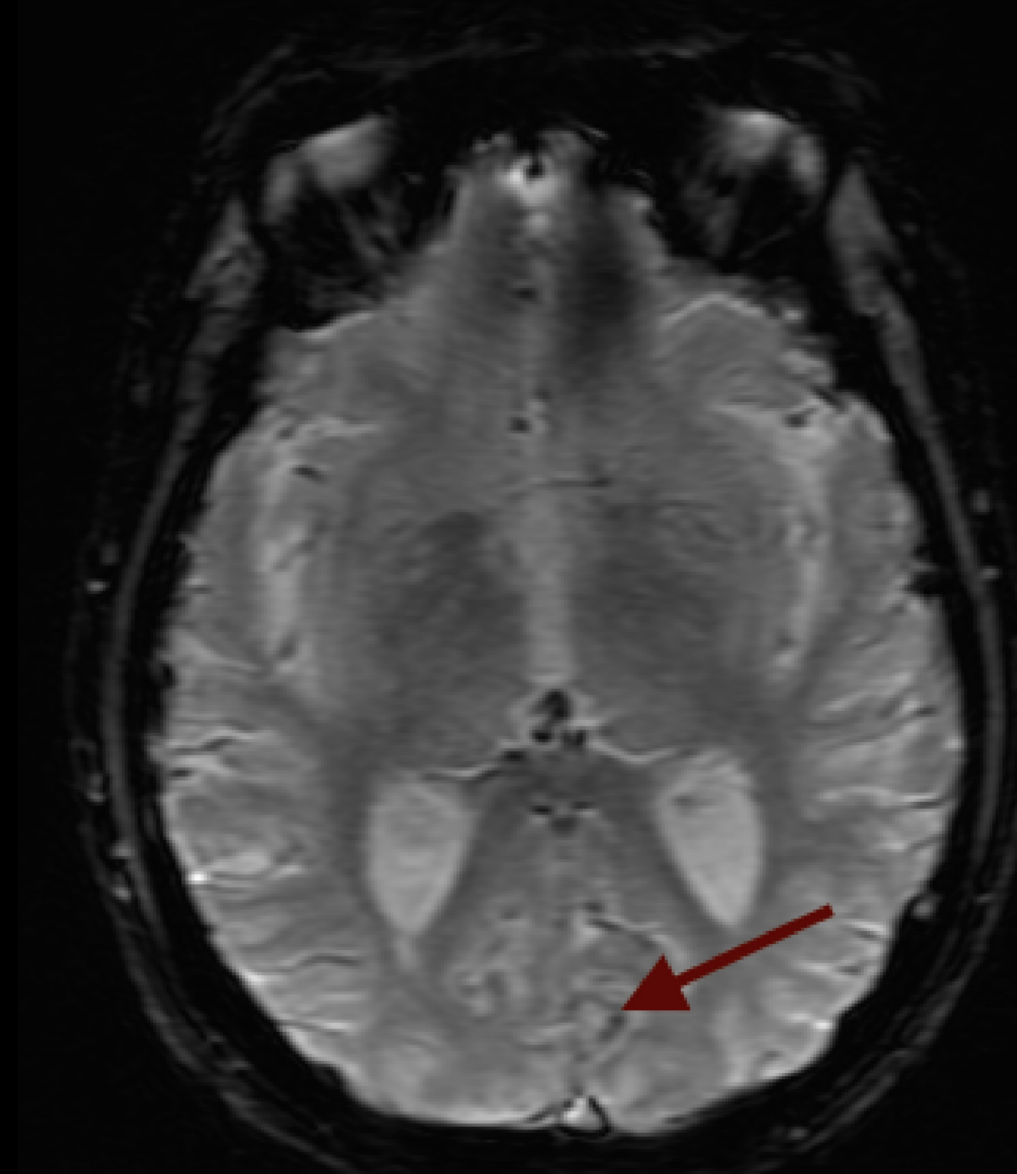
MRI: GRE demonstrating corresponding the hypointensity of the visual association cortex, V2 (red arrow) MRI: Magnetic Resonance Image; GRE: Gradient Echo Image
